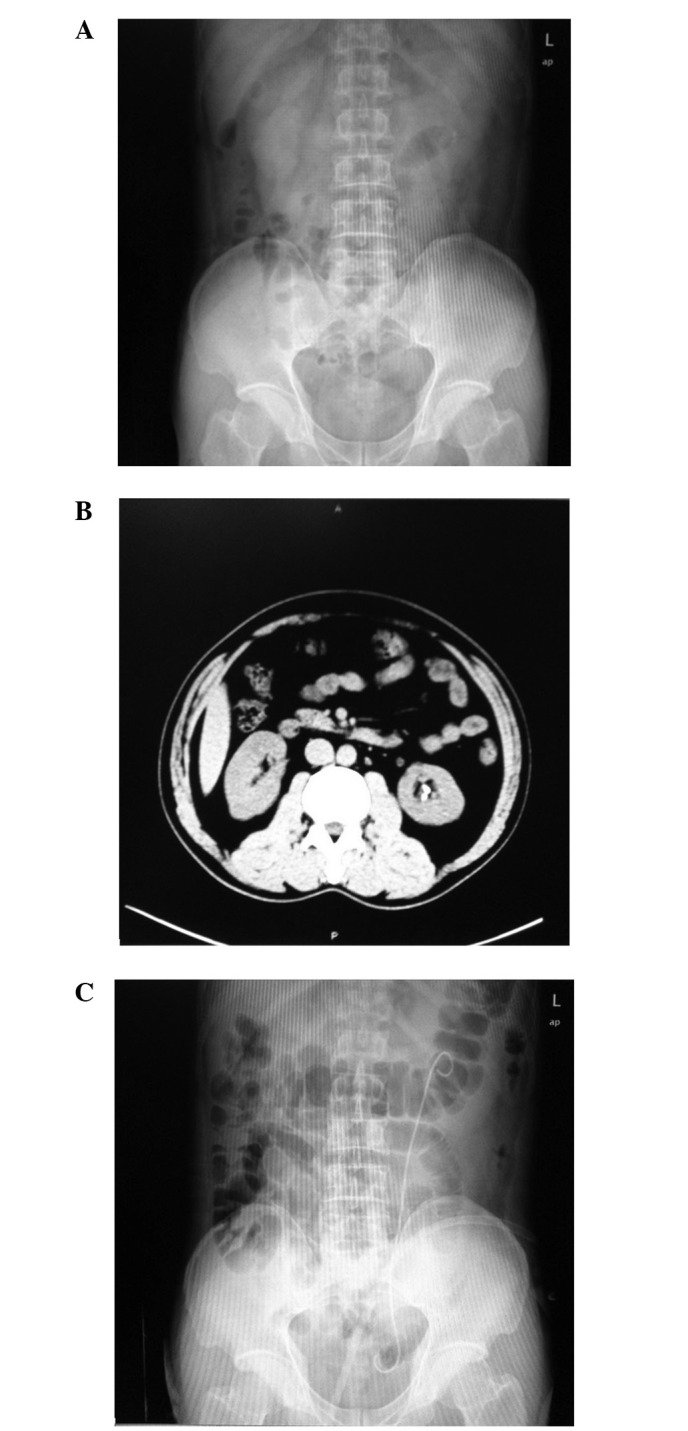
(A) Preoperative kidney, ureter and bladder (KUB) radiograph of Case 1, showing two stones in the lower calyx of the left kidney (diameter 6–7 mm). (B) Preoperative computed tomography (CT) scan showing two stones in the lower calyx of the left kidney (diameter 6–7 mm). (C) KUB radiograph of Case 1 at day 1 postoperatively, indicating that the two stones were completely removed.
